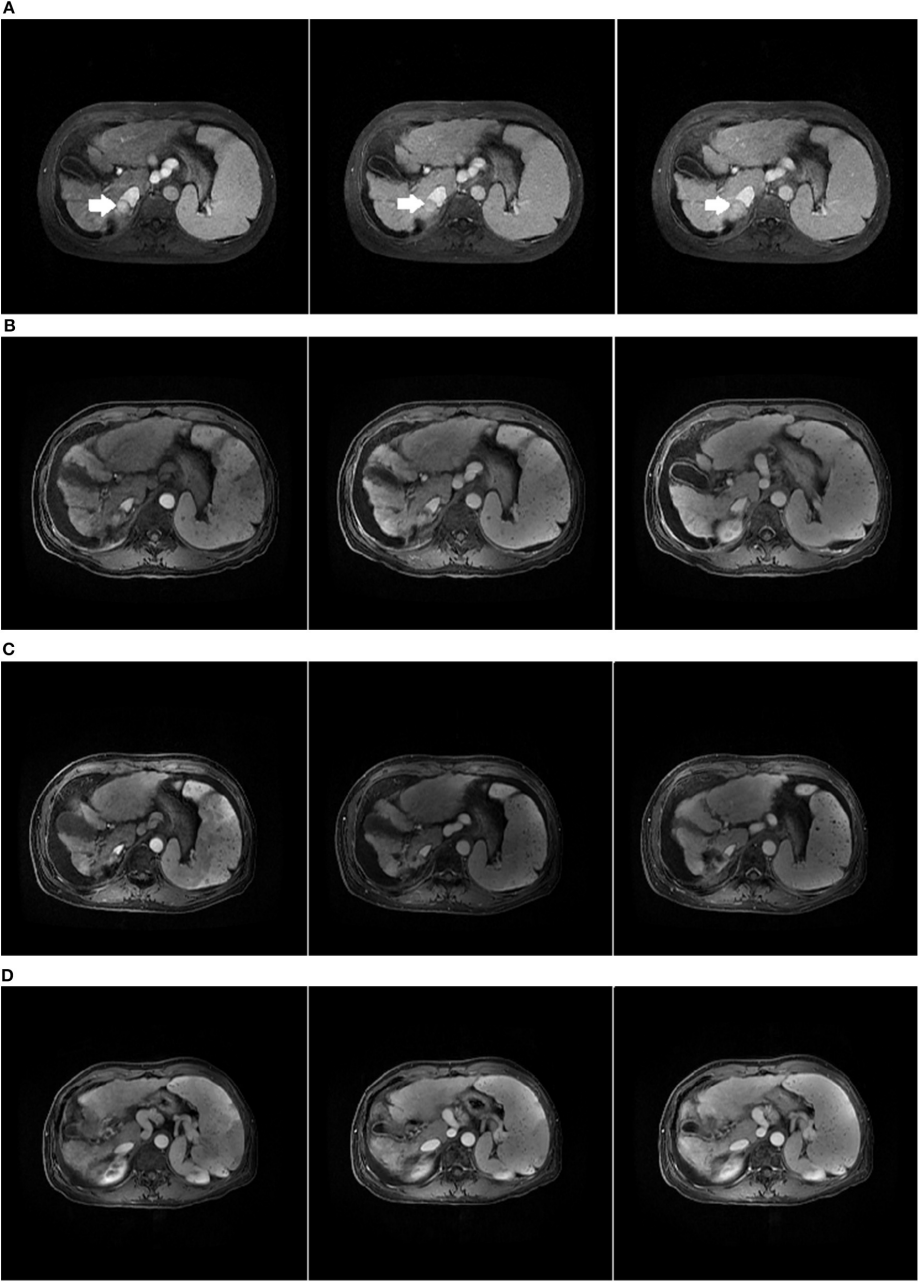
Child-Pugh C HCC patient who received CK-SBRT assessed by MRI. (A) The initial abdominal MRI scan with the primary HCC indicated by the arrow. (B) MRI scan of 3 months after SBRT. (C) MRI scan of 24 months after SBRT. The lesion in the liver disappeared. (D) MRI scan of 42 months after SBRT.
